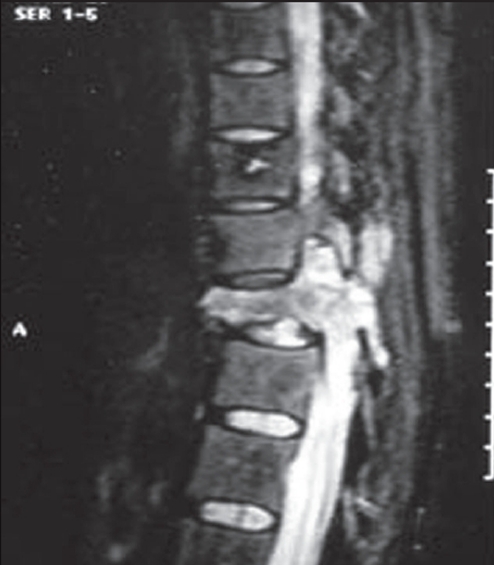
MRI appearance of a GCT in the thoracic spine. Note the lesion extending through the pedicle, affecting all three columns
